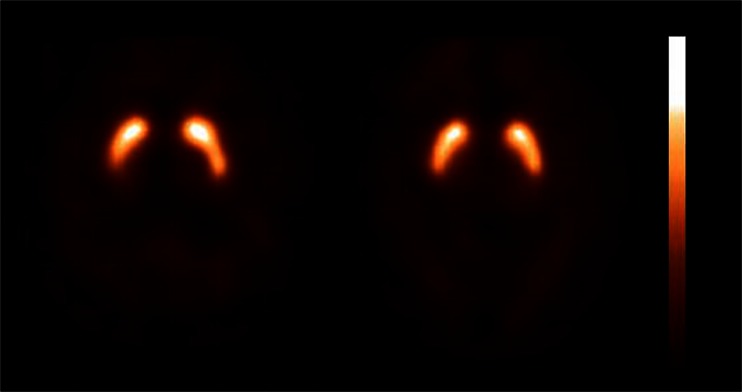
[^123^I]FP-CIT SPECT images (transversal slides at the level of the striatum) of a typical heroin-dependent patient, before (*left image*) and 2 weeks after (*right image*) an intramuscular injection with XRNT (380 mg). Note that visual analyses of the images did not show differences between the two conditions, which was confirmed by the quantitative analyses (see “Results” section)
